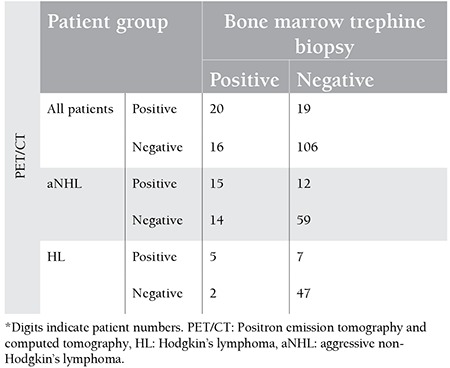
Cross-table for patients with bone marrow involvement (positron emission tomography and computed tomography vs. trephine biopsy).*
